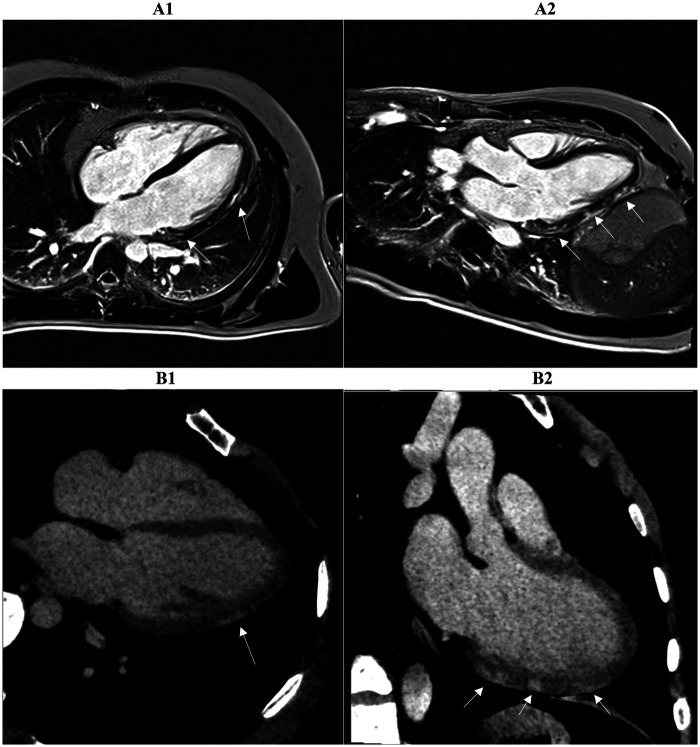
Two myocardial tissue characterization slices on CMR (A1, A2) and CCTA (B1, B2) in the same patient diagnosed with myocarditis at discharge are shown. Both the long-axis four-chamber and three-chamber views reveal intramural or sub-epicardial enhancement (arrows) with 80% segmental concordance between the imaging techniques, based on matching presence or absence of enhancement in corresponding myocardial regions. CMR, cardiac magnetic resonance; CCTA, coronary computed tomography angiography.
